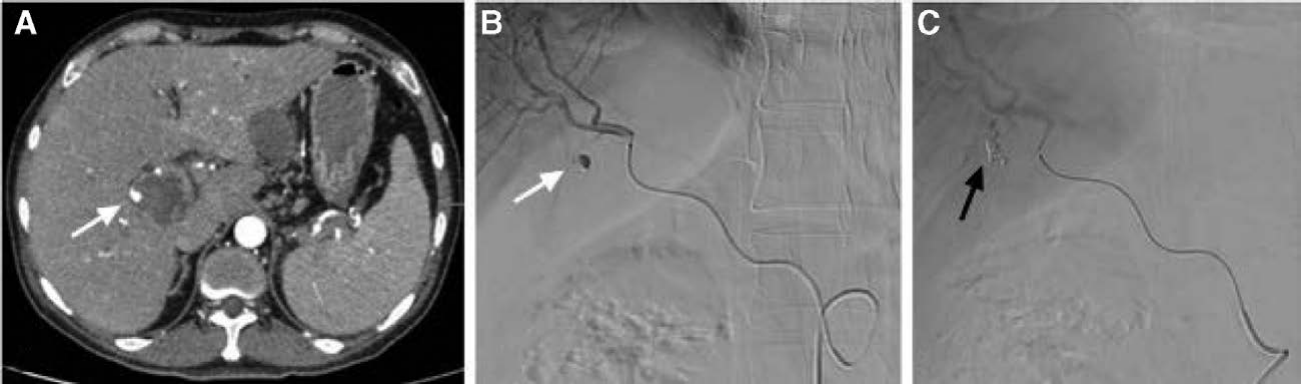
(A) Abdominal CT scan showed the presence of a right HAP with smaller diameter (white arrow), (B) Celiac angiography showed the right HAP (white arrow) in one fine branch of right hepatic artery. (C) Post embolization angiography showed occlusion of the feeding artery and pseudoaneurysm with NBCA (black arrow). CT = computed tomography, HAP = hepatic artery pseudoaneurysm, NBCA = n-butyl-2-cyanoacrylate.
